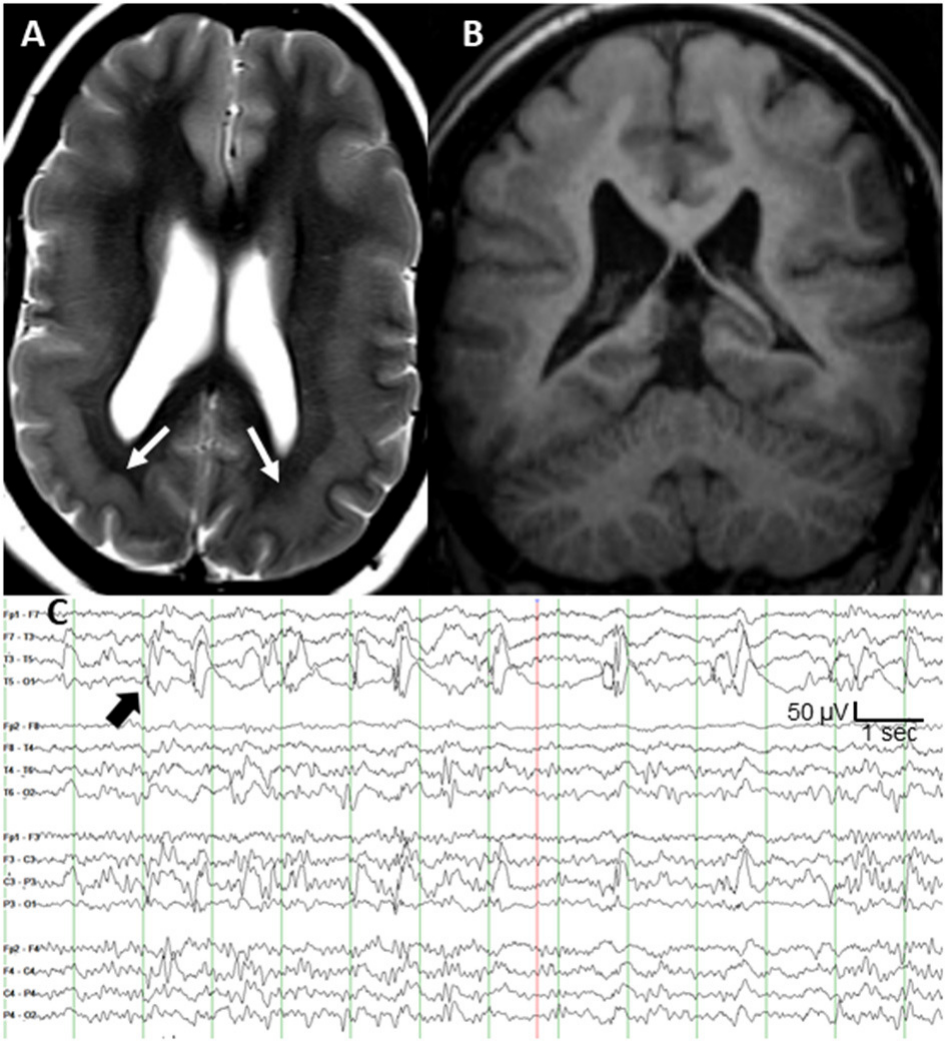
(A) T2-weighted axial MRI of bilateral posterior band heterotopia (white arrows), (B) T1-weighted coronal MRI, and (C) scalp interictal EEG with left posterior temporal spikes (black arrow).
